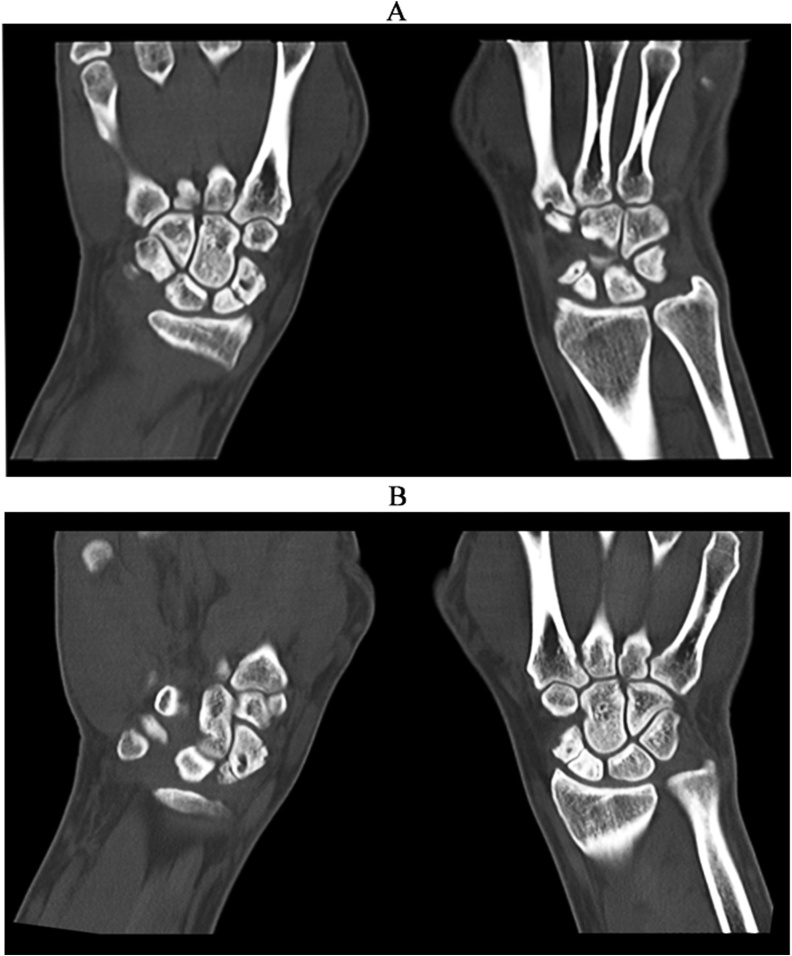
CT scan showing coronal views with bilateral scaphoid fractures.
